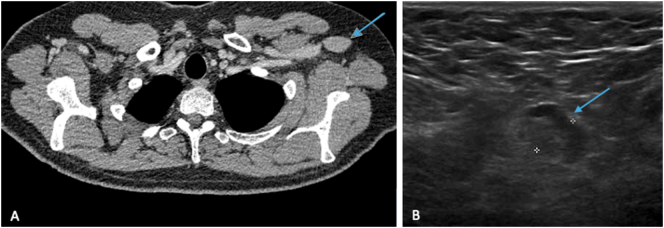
(A) CT-scan showed left axillary lymphadenopathy (arrow), two days after second COVID-19 vaccination. (B) Ultrasound showed decreased left axillary lymph node size compared to the CT-scan (arrow), six days after second COVID-19 vaccination.
